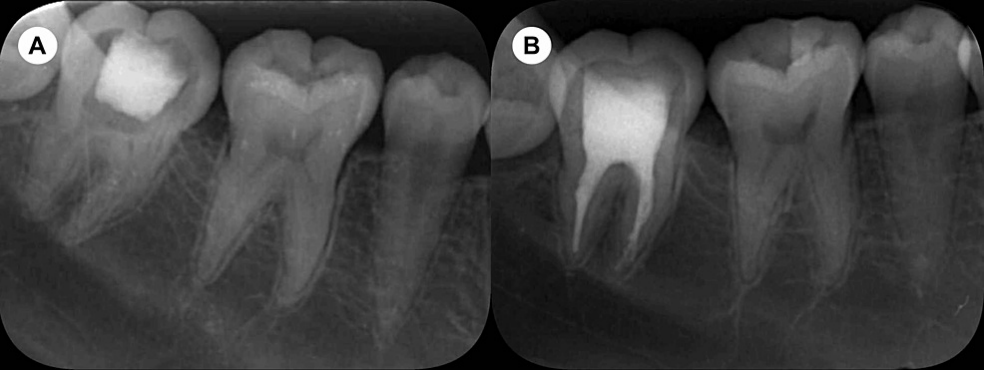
Pre-operative (A) and post-operative (B) radiographs.
